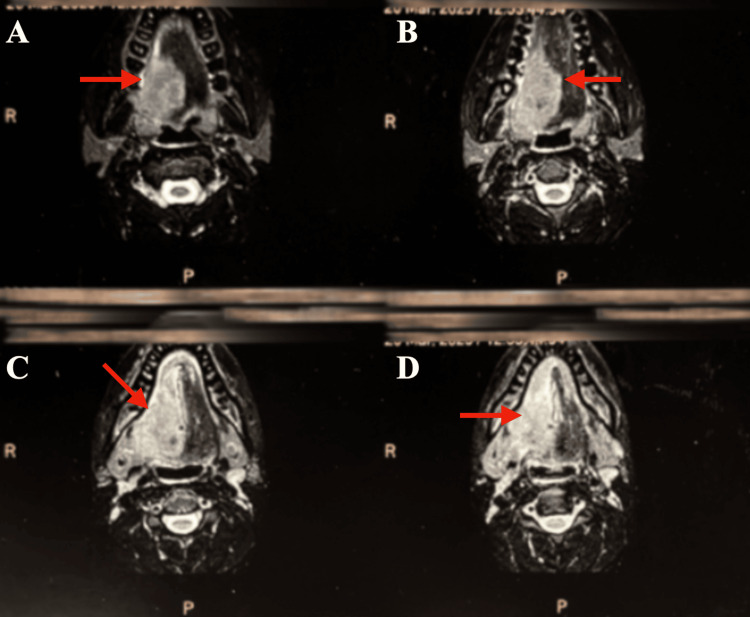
Axial MRI slices showing an infiltrative tongue mass. (A) Superior section demonstrating the upper extent of the lesion; (B) adjacent section showing that the lesion crosses the midline; (C) and (D) lower axial sections showing the lesion at its largest anteroposterior extension. MRI: magnetic resonance imaging.
